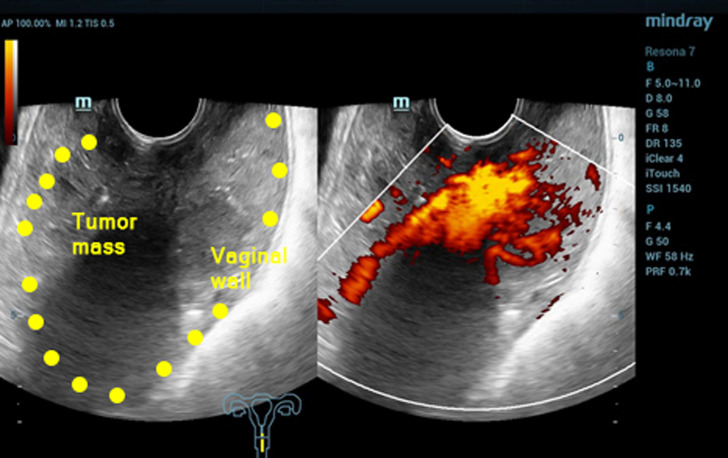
first ultrasound imaging before radiotherapy; the yellow dots indicate the tumor mass' borders
